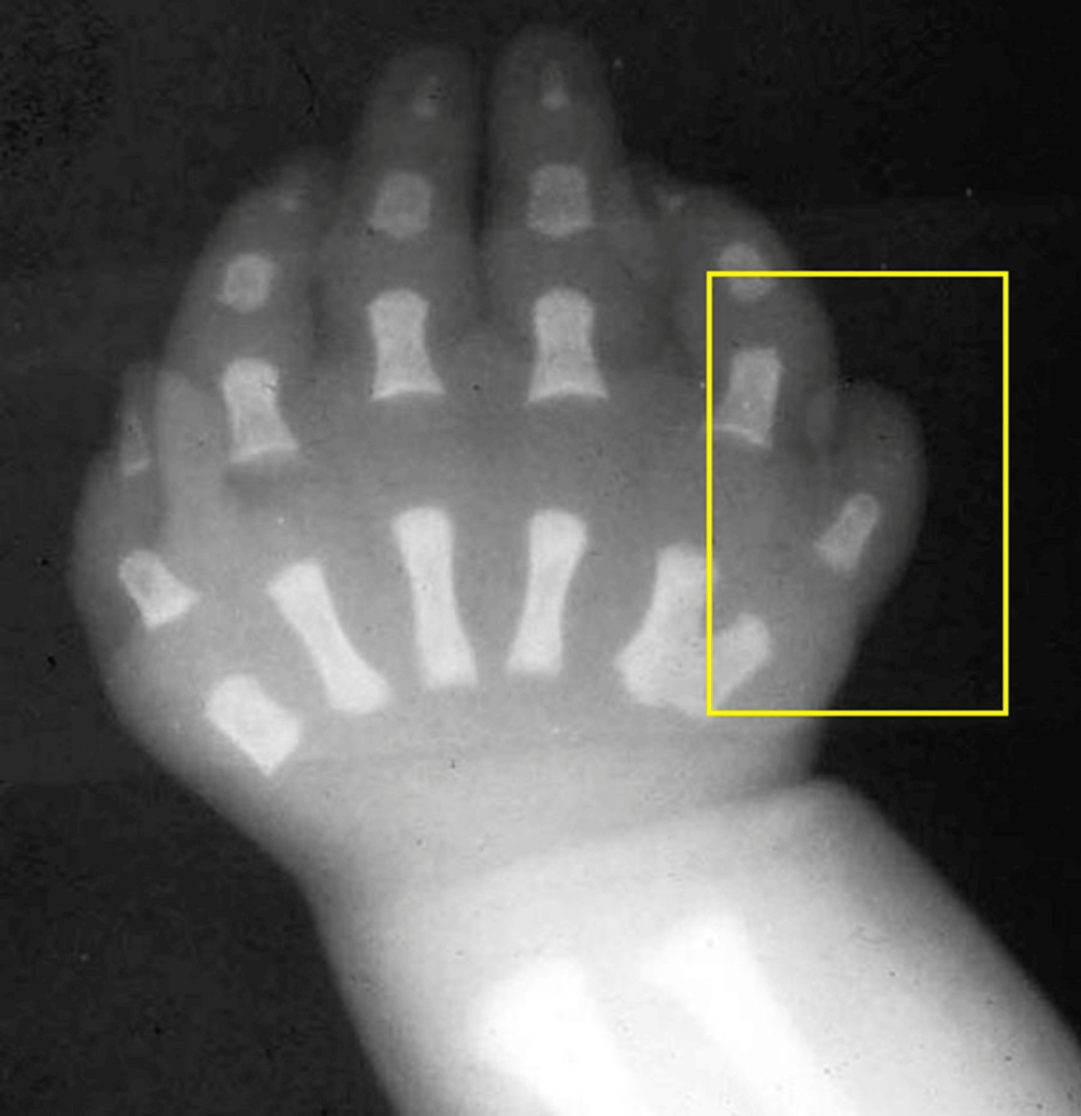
X-ray image of the patient's left hand
